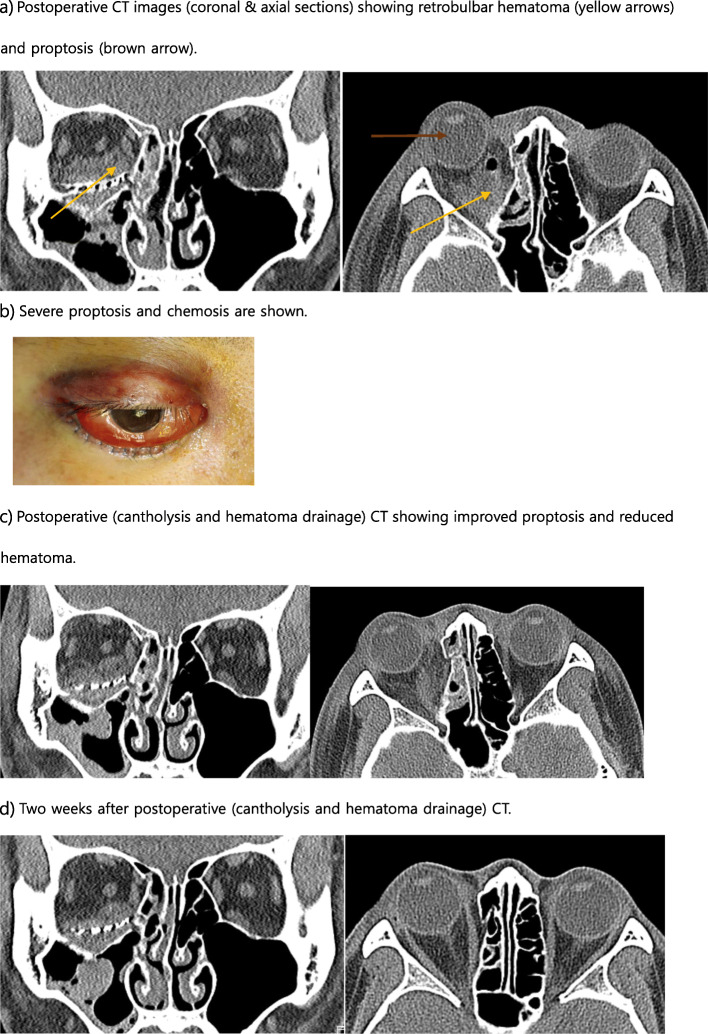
a Postoperative CT images (coronal and axial sections) showing retrobulbar hematoma (yellow arrows) and proptosis (brown arrow). b Severe proptosis and chemosis are shown. c Postoperative (cantholysis and hematoma drainage) CT showing improved proptosis and reduced hematoma. d Two weeks after postoperative (cantholysis and hematoma drainage) CT
